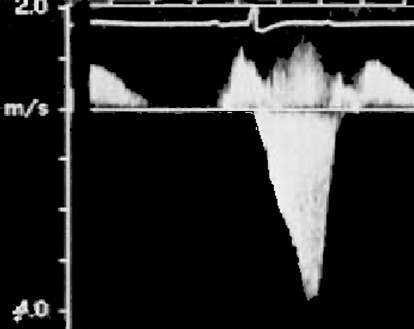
Continuous Doppler image demonstrates that the predominant gradient was subaortic and not simply midventricular.
